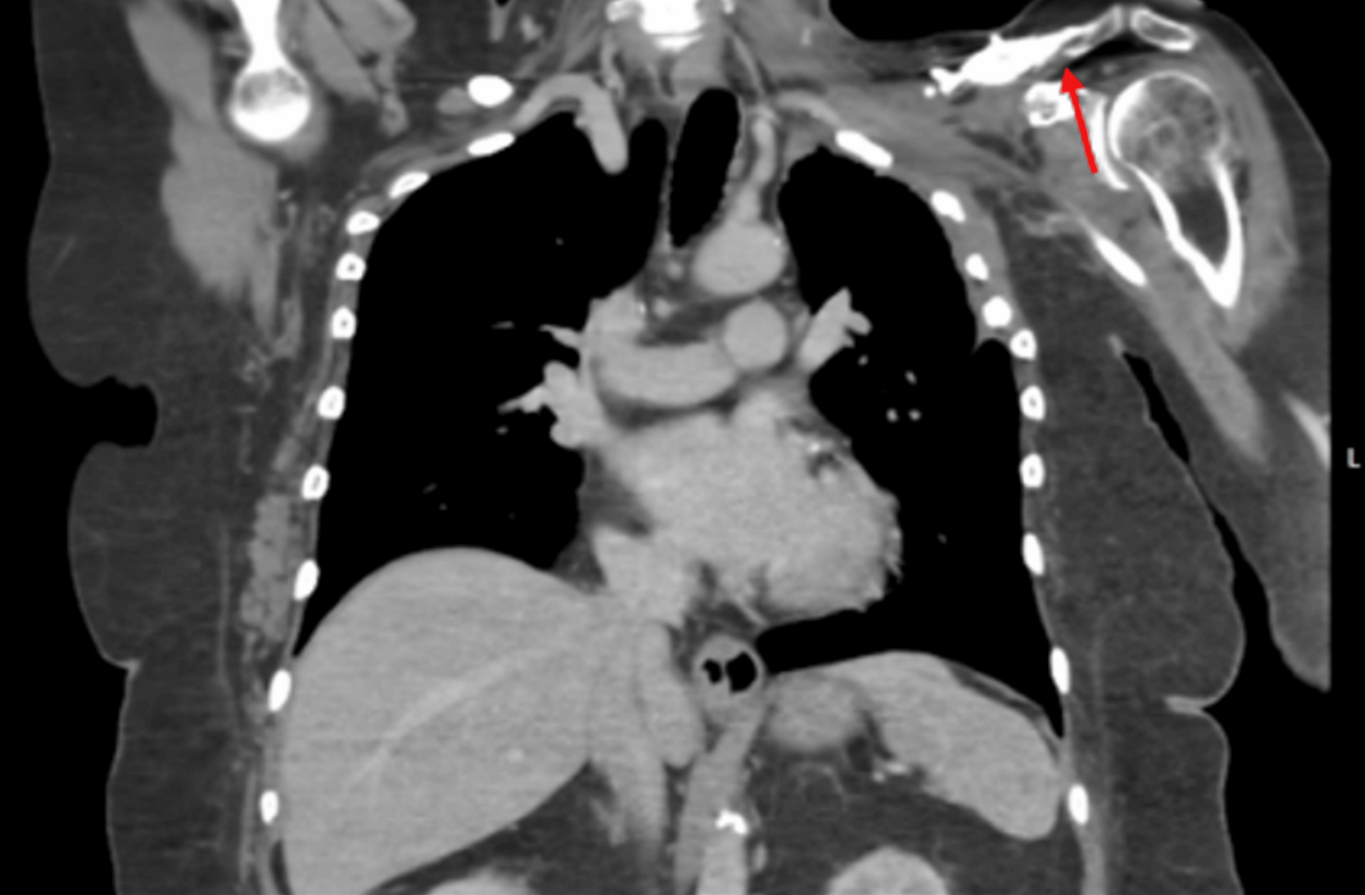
Coronal computed tomography showing soft tissue thickening and bony changes of the left scapula clavicle and humerus.
